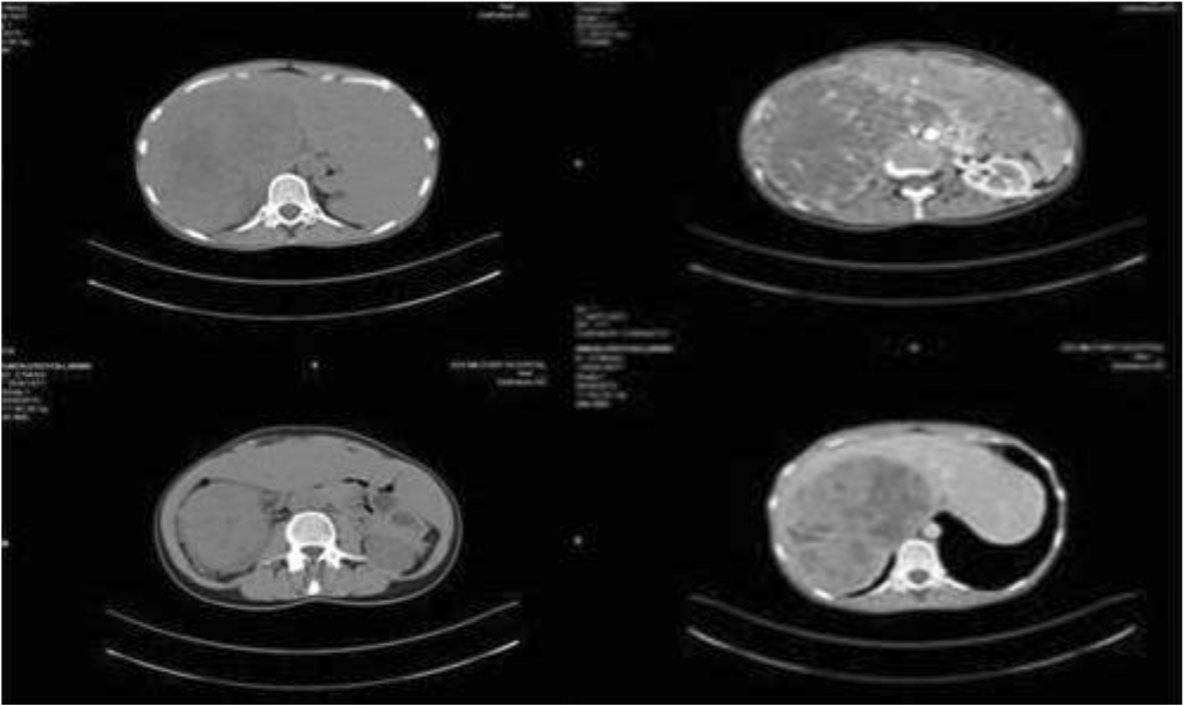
Computed tomography appearance of giant adrenocortical carcinoma
